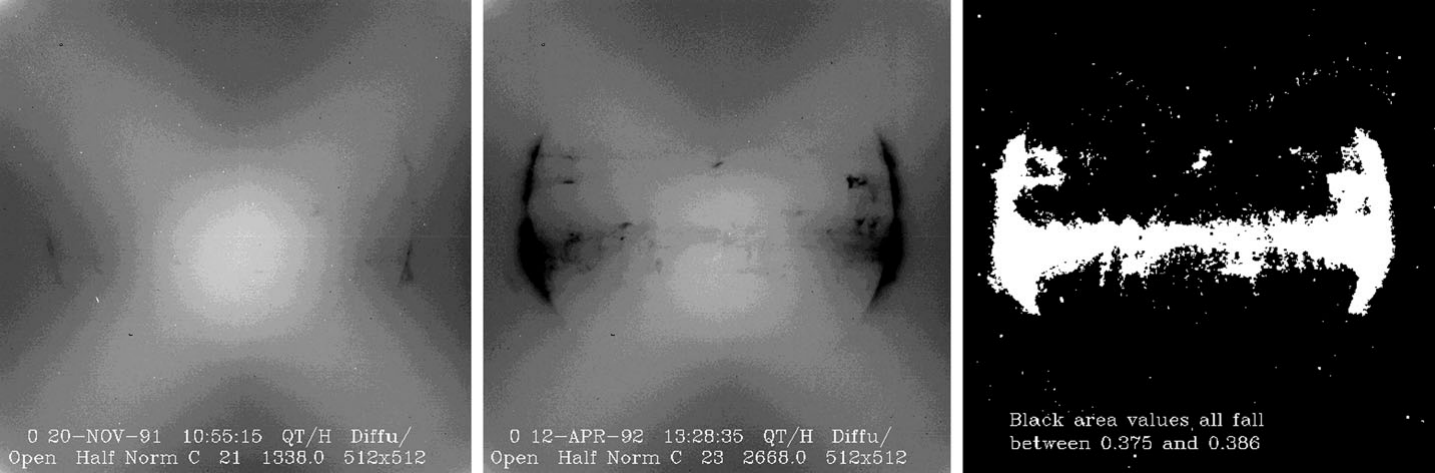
SXT diffuser images obtained 20 November 1991 (left) and 12 April 1992 (center). X-ray damaged area is evident in the center (later) image. In the rightmost panel the black portion shows the area of the CCD in which the signal decrease is very uniform, falling between 61.4 and 62.5 %.
